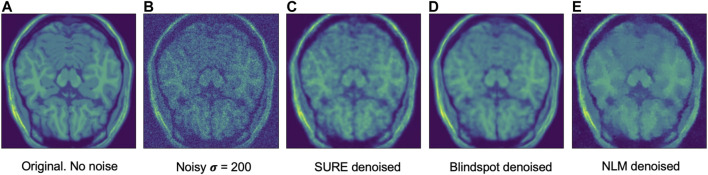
Example of denoised brain MRI for σ=200. The example image is the middle slice from one of the subjects. In this case, this is the PSNR for every method for this particular subject. (A) Original image, no noise added—(B) Noisy image—(C) SURE PSNR = 29.610—(D) Blindspot PSNR = 30.904—(E) NLM PSNR = 26.616.
